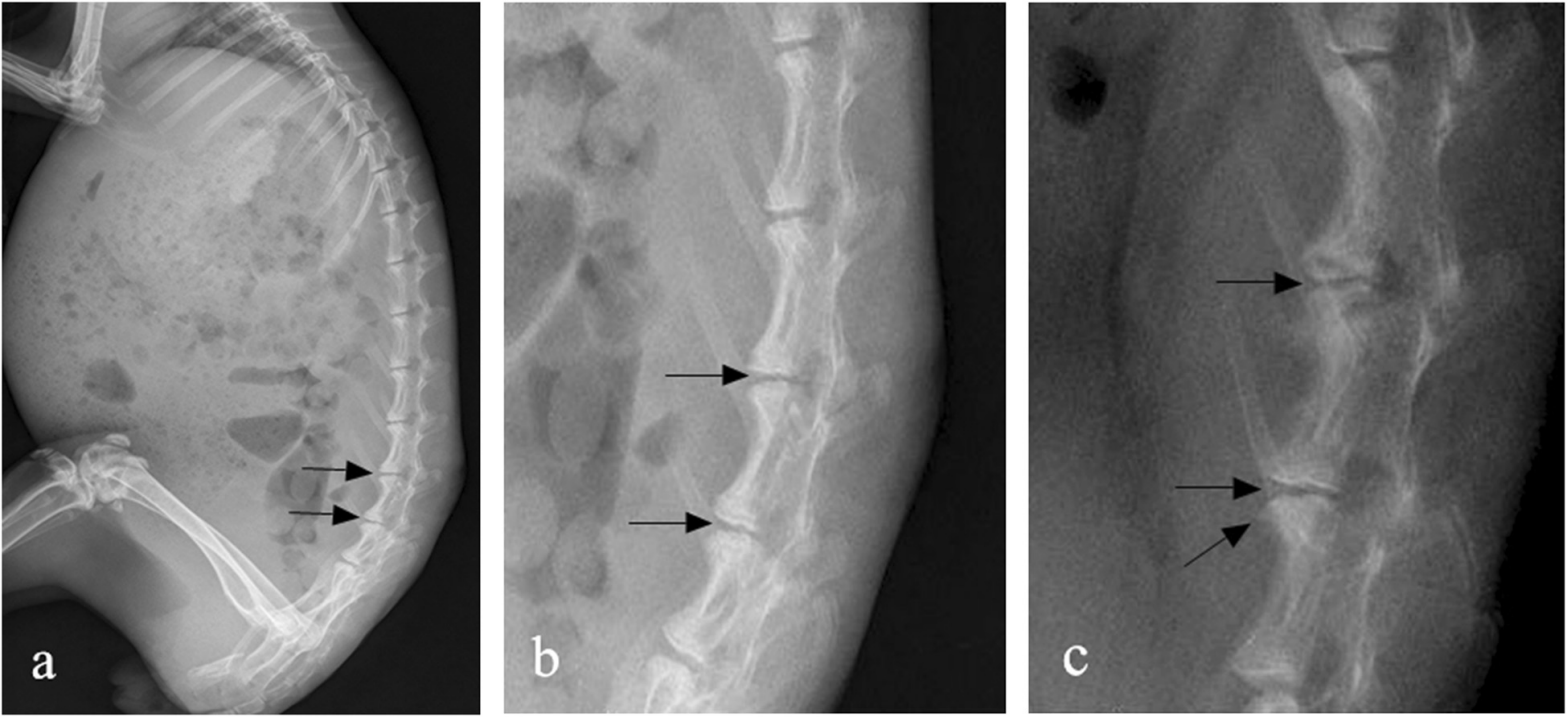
Lateral radiographs showing L4–5 and L5–6. (a) Reduced disk height of L4–5 and L5–6 on lateral plain radiographs at 4 weeks after stabbing (black arrows). (b) Neither osteophyte formation nor endplate thickening were observed during this period, only reduced disk height is apparent (original magnification × 2.5). (c) Further loss in disk height was seen at 8 weeks after stabbing (black arrows). Early osteophyte formation (oblique arrow) was observed in this period, but endplate thickening still did not appear (original magnification × 2.0).
